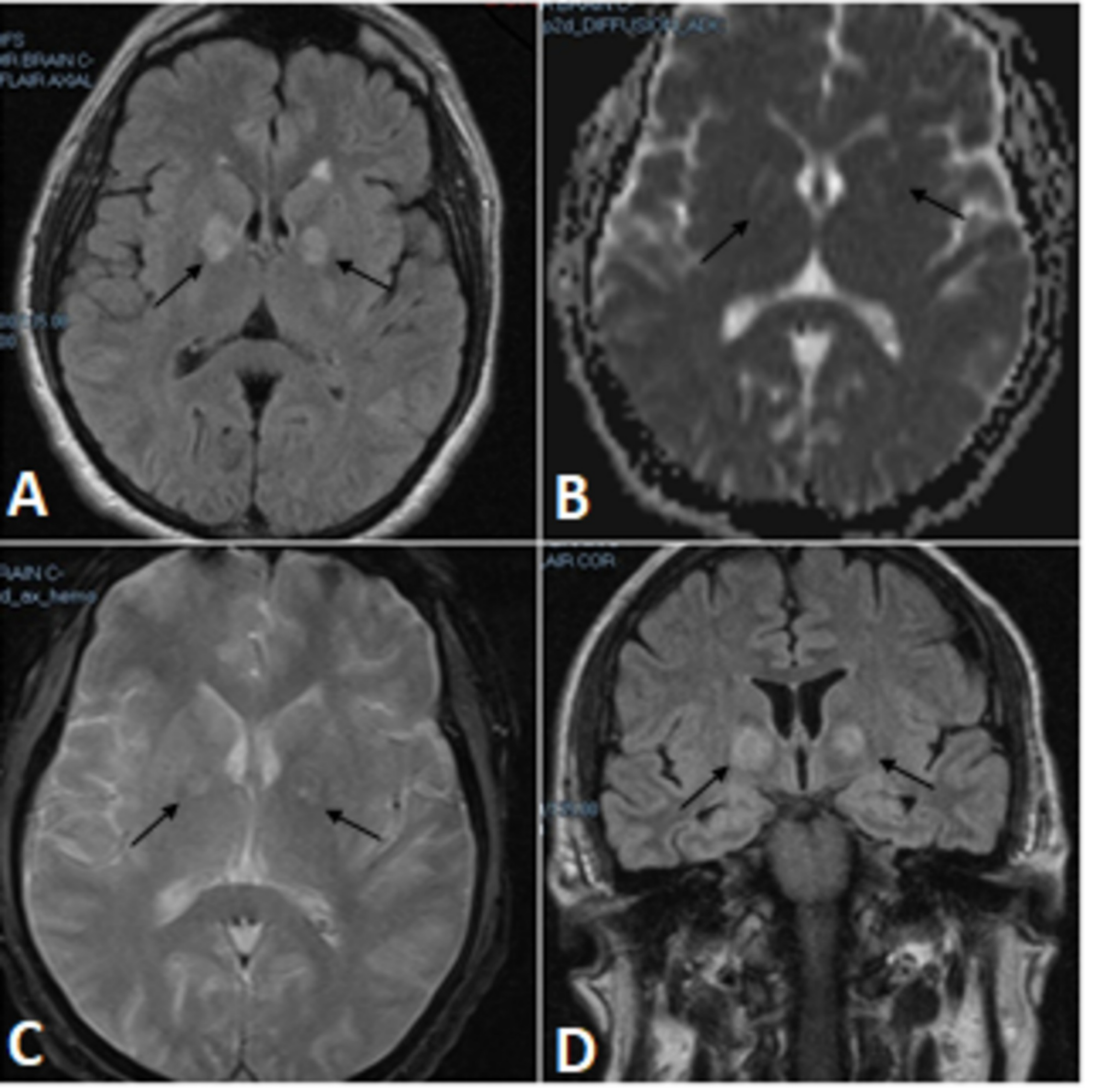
Magnetic resonance imaging (MRI) of the brain. MRI of the brain revealed two areas of increased T2/FLAIR signal within the medial aspect of both basal ganglia, measuring 16 mm in the right and 12 mm on the left involving each globus pallidus and the genu of the internal capsule.
